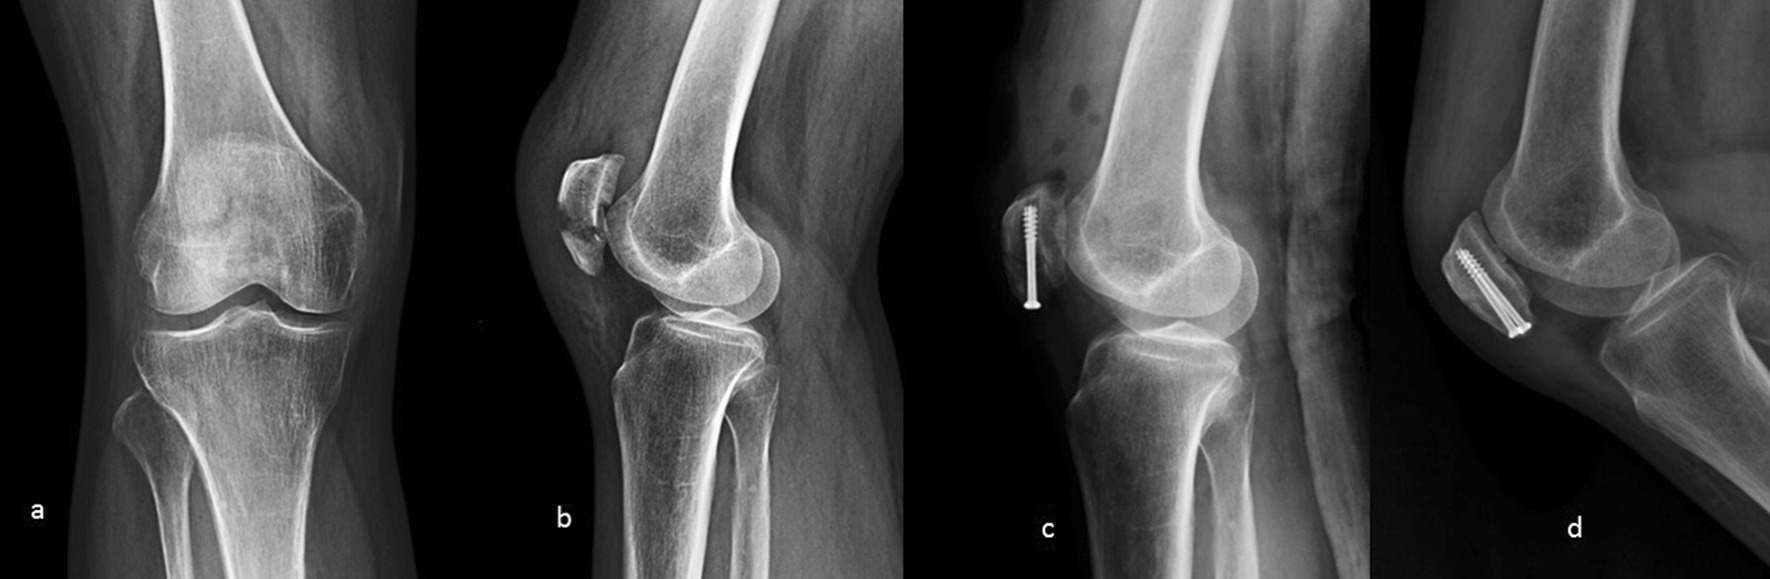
Plain radiographs of the patient (number 12). a before surgery; b before surgery; c at 1 day postsurgery; d at 1 year postsurgery
